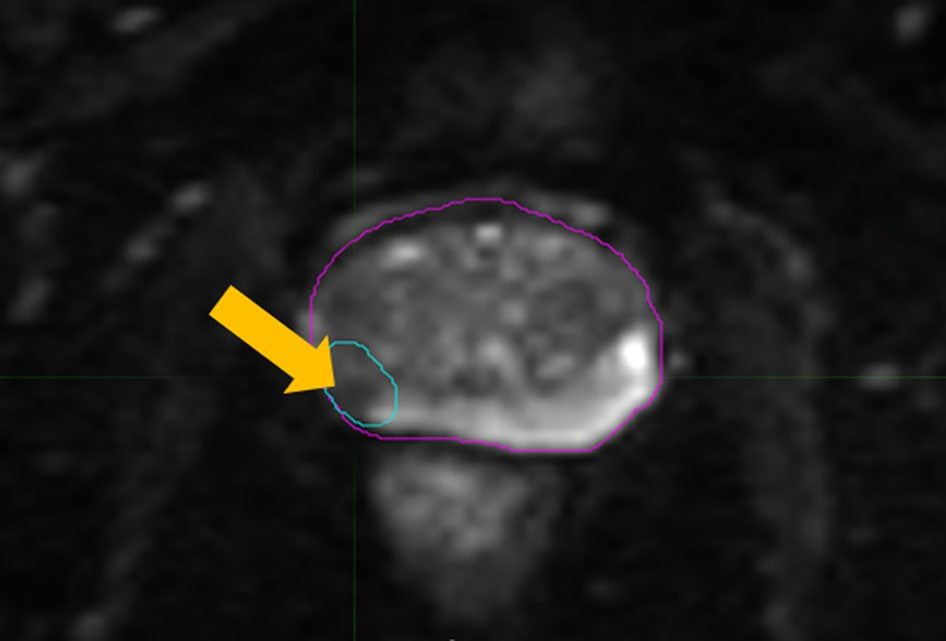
Diffusion-weighted imaging (DWI) of an axial midgland plane with high *b* value ADC map. Areas of higher water diffusion rates appear brighter on T2 imaging. Significant cancers may present with restricted diffusion rates and are seen as areas of hypointense signal on the ADC map (*arrow*). The focal lesion is contoured for clarity
